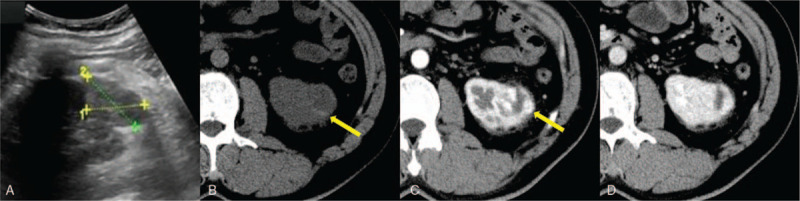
A 45-year-old man with a round mass in the left kidney diagnosed as hemangioblastoma. (A) Ultrasonography revealed a 37 × 42 mm heterogeneous echo mass inside the lower pole of the left kidney that had an irregular shape and a distinct margin. (B) Axial CT images of the precontrast phase of the abdomen showed a round soft tissue mass in the lower pole of the left kidney, and a small high-density shadow could be seen in the periphery of the mass (yellow arrow), which suggested a hemorrhage. (C) In axial CT images of the corticomedullary phase, the mass showed peripheral nodular enhancement (yellow arrow), similar to that of the abdominal aorta at the same level. (D) Axial CT images of the nephrographic phase showed slight progressive centripetal enhancement.
